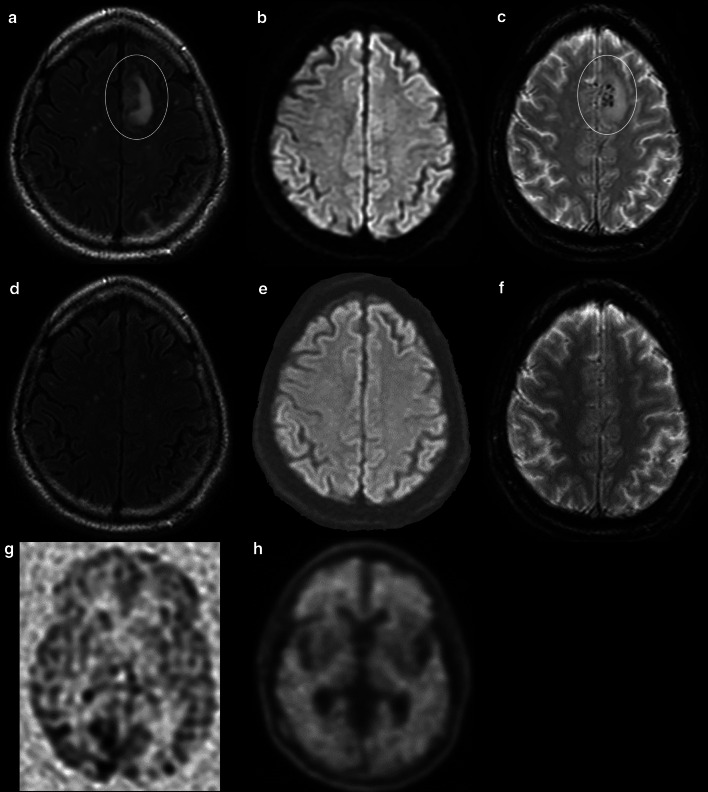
A 67-year-old man with AD on anti-Aβ monoclonal antibody trial. T2 FLAIR image shows hyperintensity in the parasagittal left frontal lobe (oval in a), with no evidence of hyperintensity on DWI (b), and associated subcortical microhemorrhages (oval in c). None of these abnormalities were present prior to receiving antibody therapy (d–f), consistent with ARIA with edema and hemorrhage. Arterial spin labeling demonstrates hypoperfusion to the parietal and frontal lobes (g), with corresponding diffuse cortical uptake on florbetapir PET/CT typical of AD (h)
